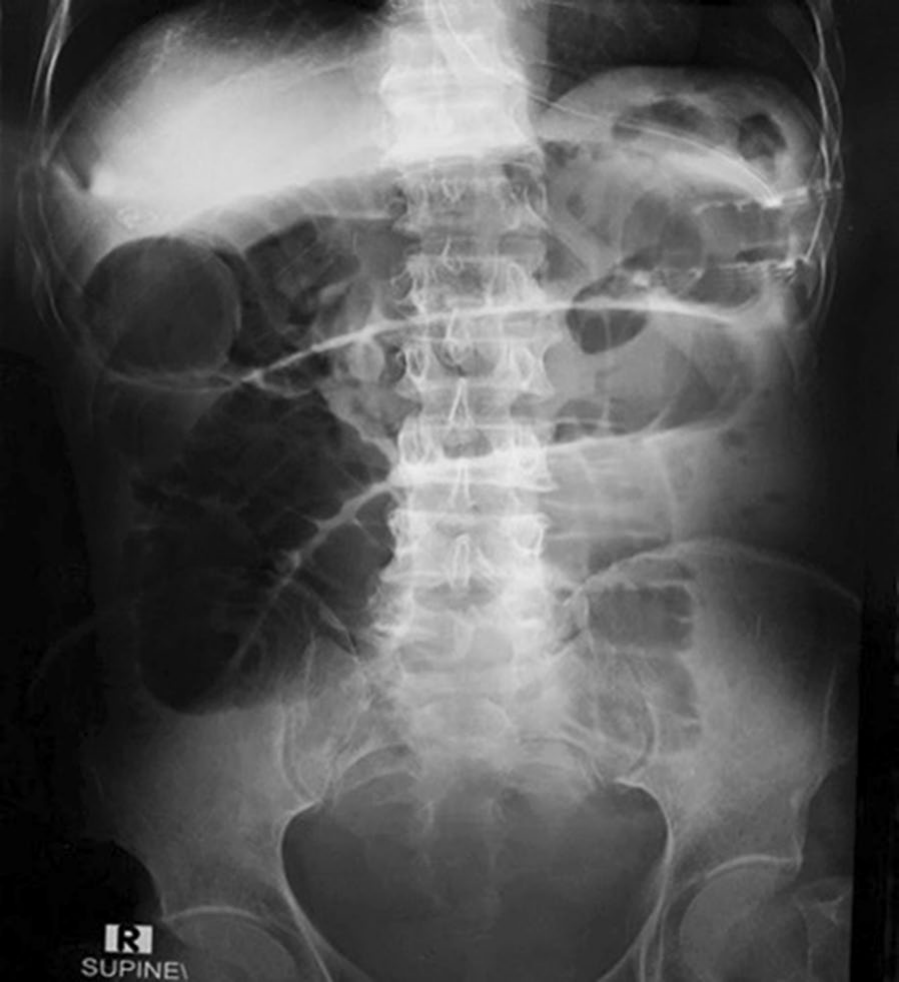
Supine abdominal X-ray shows multiple dilated small bowel loops with absent rectal gas shadows
